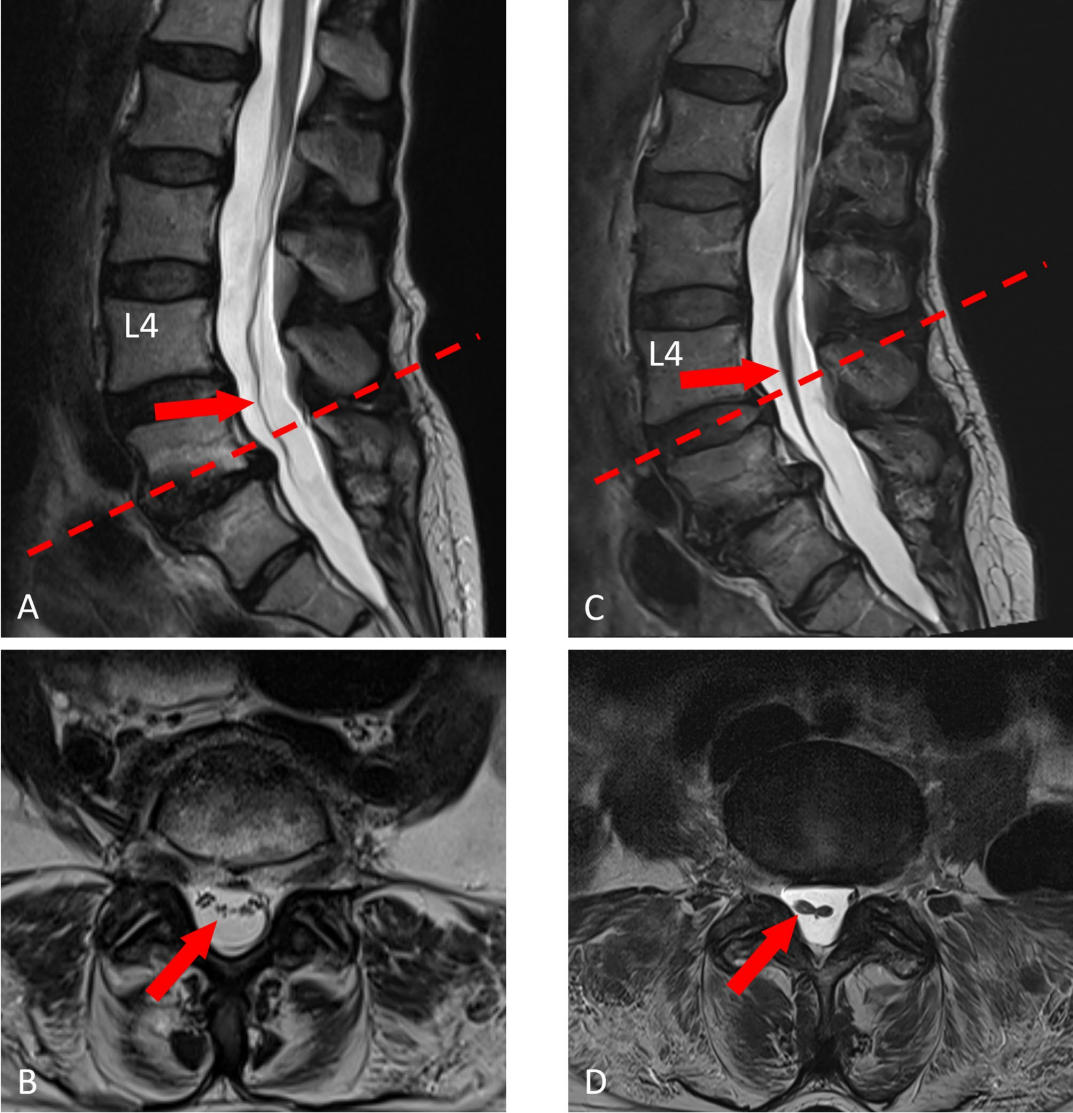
MRI of the lumbar spine of Case 1 one day (A, B) and two years (C, D) after the epidural steroid injection with sagittal (A, C) and transverse (C, D) T2-weighted sections. The MRI one day after the injection shows an anterior displacement of the cauda nerve roots from the L3 to S1 level (arrows in A and B), suggesting adhesions of the nerve roots to the dural sac and possibly an early sign of arachnoiditis. The follow-up MRI two years after the injection shows a clumping of the cauda nerve roots to a central cord-like structure (arrows in C and D) and peripheral adherence of the nerve roots to the dural sac distally (not shown), representing a typical finding after arachnoiditis. Note: the dashed line in A describes the orientation of the transverse section B at level L5/S1, the dashed line in C describes the orientation of the transverse section D at the level L4/5
